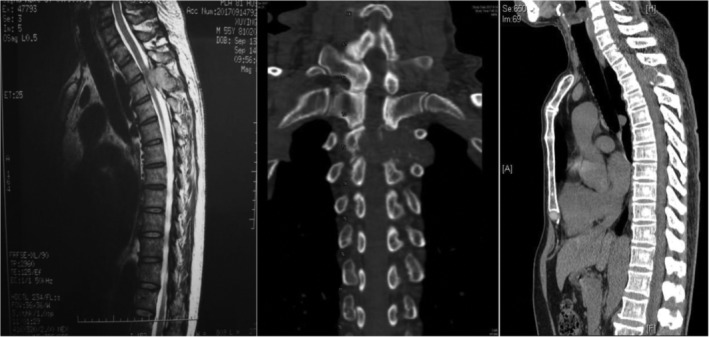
Preoperative MRI and CT scan.
